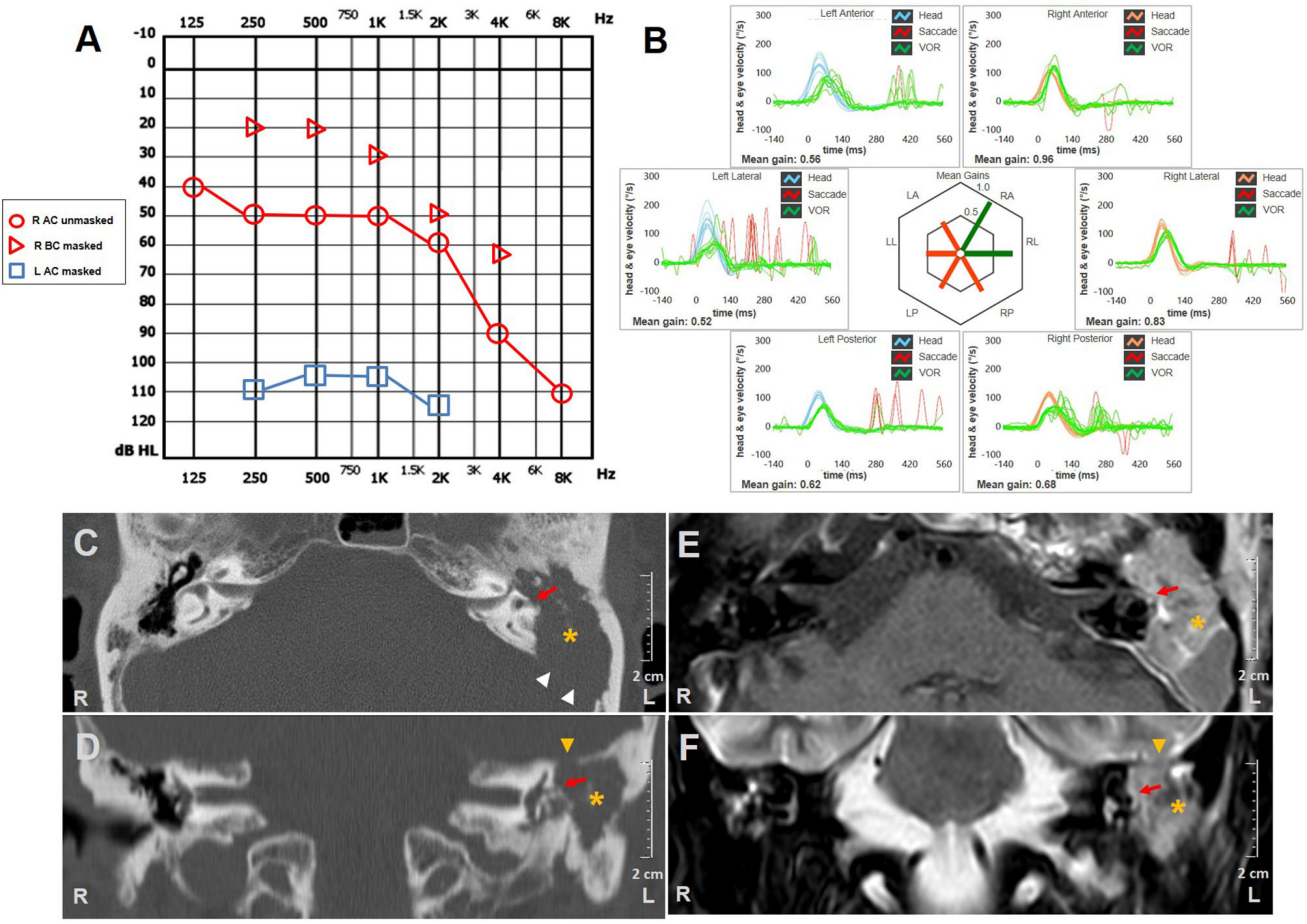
Instrumental and radiological data of case one. (A) Audiometry showing mixed HL on the right side and profound HL on the left. (B) vHIT measurements. Blue lines represent head impulses exciting left SCs, orange lines correspond to impulses for right SCs, green lines represent eye movements induced by the activation of VOR following each impulse and red lines correspond to corrective saccades. Mean value of VOR-gain (eye velocity/head velocity) is reported for each SC. The hexagonal plot in the center of the figure summarizes mean VOR-gains for each SC; normal gains are shown in green and deficient gains are in red. A global canal hypofunction on the left and a slight reduction of the VOR-gain for contralateral PSC with overt saccades could be clearly observed. Axial (C) and coronal (D) images of temporal bones CT scans completed with axial T1-weighted (E) and coronal T2-weighted (F) gadolinium-enhanced brain MRI showing soft tissue density (*yellow asterisks*) within left external/middle-ear and mastoid cavity disrupting surrounding structures. Ossicular chain is not detectable. Bony erosion areas at the posterior fossa (*white arrowheads*) and middle fossa floor (*yellow arrowheads*) with dural infiltration are highlighted. Otic capsule erosion at the left HSC is indicated with *red arrows*. AC, air conduction; BC, bone conduction; CT, computed tomography; HL, hearing loss; HSC, horizontal semicircular canal; L, left; LA, left anterior; LL, left lateral; LP, left posterior; MRI, magnetic resonance imaging; PSC, posterior semicircular canal; R, right; RA, right anterior; RL, right lateral; RP, right posterior; SC, semicircular canal; vHIT, video-head impulse test; VOR, vestibulo-ocular reflex.
